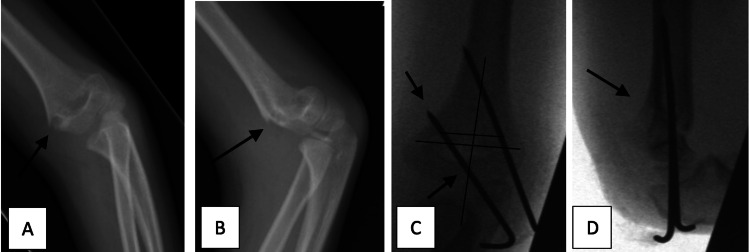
Radiographic example of adequate fixation using lateral K-wires technique (A, B) Preoperative AP and lateral radiographs with displaced supracondylar fracture. (C, D) Intra-operative anteroposterior and lateral radiograph showing bi-cortical, bi-columnar, divergent lateral wire fixation and separation of wires >1/3 circumference of the humerus at the fracture site.
